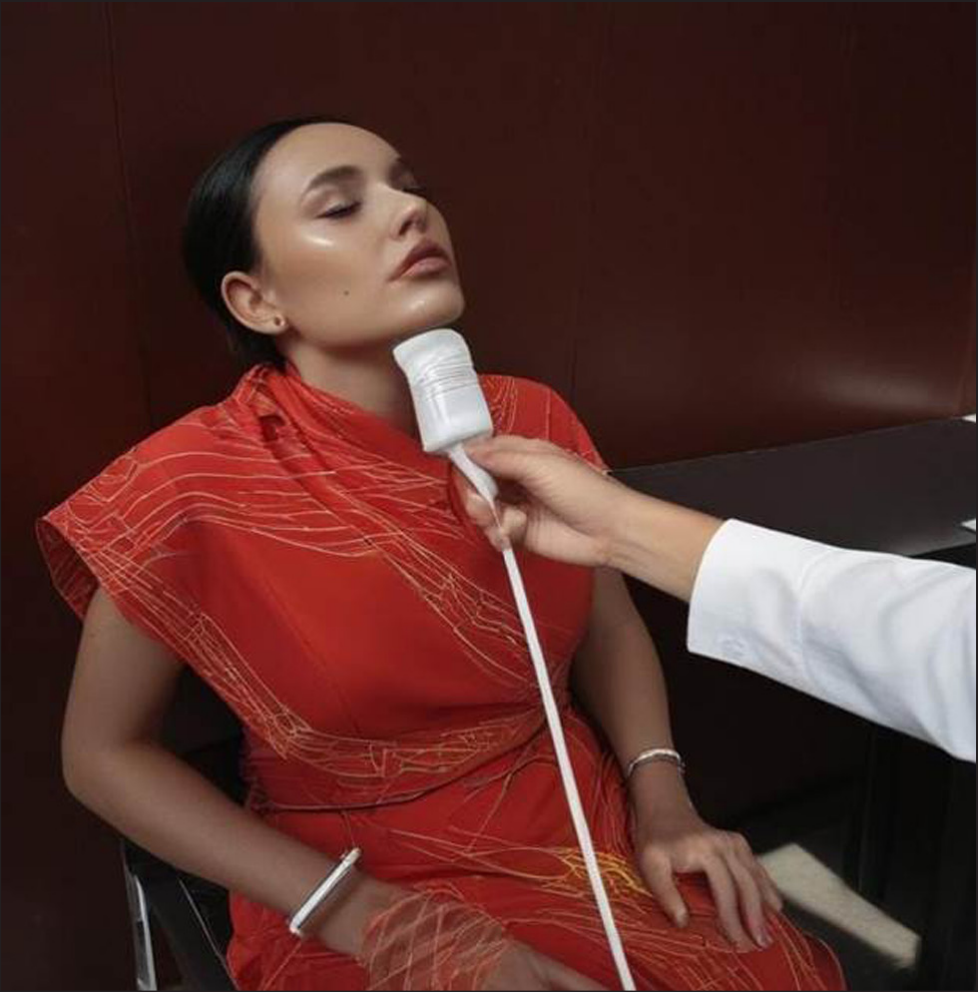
Shows the ultrasonography technique of submental view with the linear probe.
